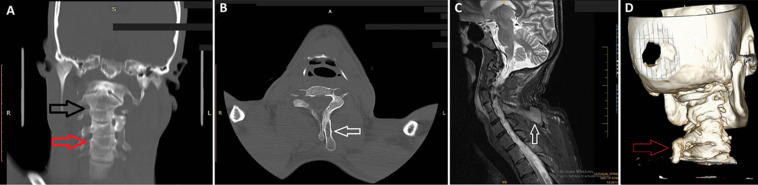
A) computed tomography scan bone window coronal image showing congenital fusion at two levels of the cervical spine, C2-C3 (black arrow) and C3-C4 and C5 (red arrow); B) computed tomography axial bone window image; C) sagittal STIR image (open arrow) showing an osseous overgrowth at the level of C3-C4 involving the left lamina with extension into the paravertebral region suggestive of omovertebral bone and two spinous process; D) computed tomography reformatted image showing omovertebral bone
